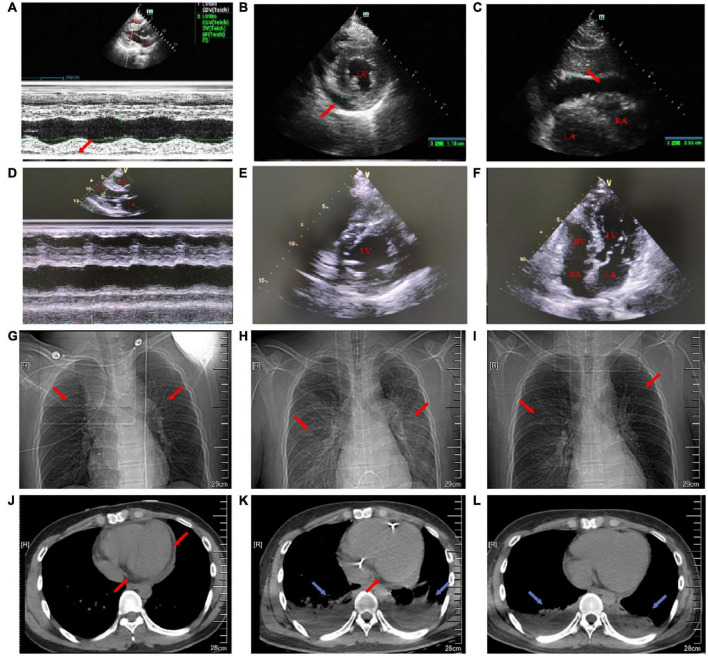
Transthoracic echocardiography on the first day and the 18th day of admission. Chest X-ray and chest CT images on the first, eighth, and 15th day of admission. (A) On the first day of admission, the maximum anterior–posterior diameter of the posterior dark area of the left ventricular posterior wall on the long-axis view of transthoracic echocardiography was 0.8 cm (red arrow). (B) On the first day of admission, the maximum anterior–posterior diameter of the dark area of the lower wall of the left ventricle on the short-axis view of transthoracic echocardiography was 1.2 cm (red arrow). (C) On the first day of admission, transthoracic echocardiography showed that the anterior–posterior diameter of the dark area under the xiphoid process was 2.5 cm (red arrow). (D) On the 18th day after admission, the pericardial effusion on the long-axis view of transthoracic echocardiography was less than that before. (E) On the 18th day after admission, the pericardial effusion on the short-axis view of transthoracic echocardiography was less than that before. (F) On the 18th day of admission, transthoracic echocardiography four-chamber view. (G) On the first day of admission, a chest X-ray showed a few fibrous foci in both the lungs (red arrow). (H) On the eighth day of admission, the chest X-ray showed inflammation in the upper lobe of the left lung and the middle lobe of the right lung (red arrow). (I) On the 15th day of admission, the chest X-ray showed a little inflammation of the left upper lung (red arrow). (J) On the first day of admission, the cross-section of the chest CT soft-tissue window showed that the pericardial fat space was blurred and there was a large amount of pericardial effusion (red arrow). (K) On the eighth day of admission, the cross-section of the chest CT soft-tissue window showed bilateral pleural effusion with bilateral lower lung insufficiency (blue arrow) and a small amount of pericardial effusion (red arrow). (L) On the 15th day of admission, the cross-section of the chest CT soft-tissue window showed bilateral pleural effusion and bilateral lower lung insufficiency (blue arrow).
